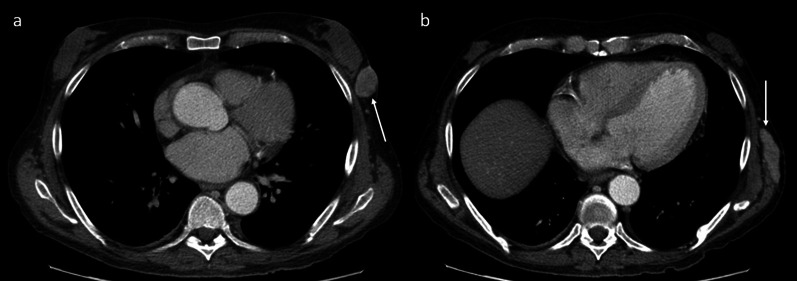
Soft tissue recurrence. Axial contrast-enhanced CT images on the arterial phase (a, b) exhibit recurrences of HCC involving the soft tissues of the left thoracic wall (arrows). These lesions show the typical pattern of the primary HCC, characterized by hyperenhancement on the arterial phase
